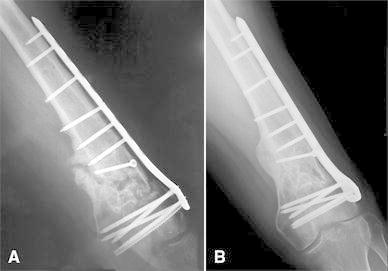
Male 46 years. a AP view before second surgery shows a non-union. b Radiograph at last follow-up shows fracture healing
